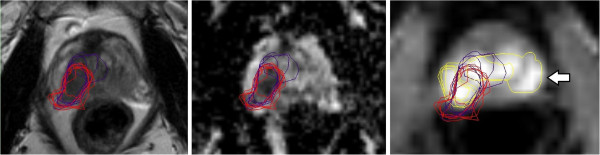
(Patient No.1). Transversal slices through the middle prostate gland; left = T2w-, middle = DWI-, right = DCE-sequence. Left and middle picture show T2w (violet contour) and DWI (red contour) based GTV-delineation respectively. On the right image additionally DCE (yellow contour) based GTV-delineation; the arrow indicates delineation of non-specific enhancement by two observers in the left gland symmetric to the right side.
